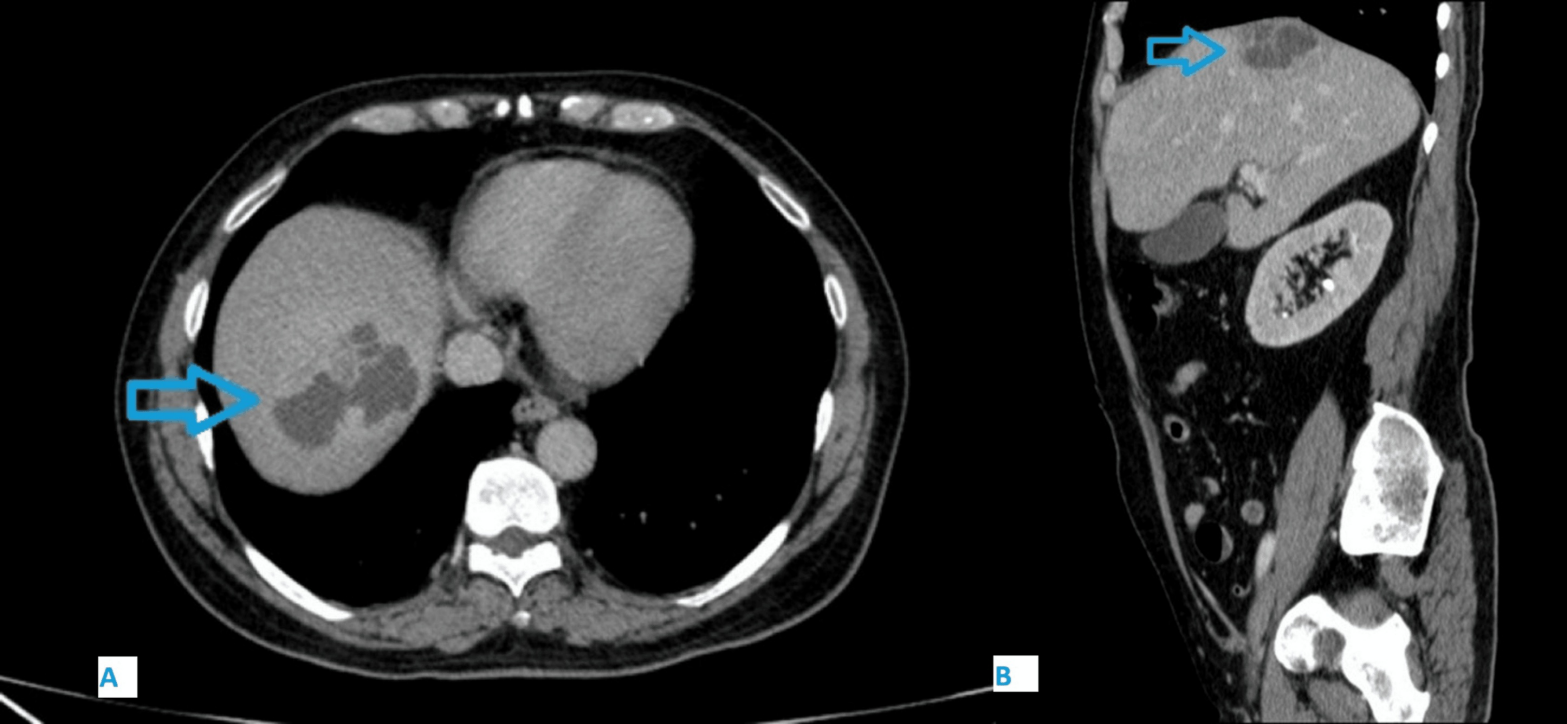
Abdominal CT images showing a liver lesion. (A) Axial view and (B) sagittal view CT of the abdomen reveals an irregular fluid-density lesion within the liver, demonstrating rim enhancement and a thin outer ring of hypoattenuation, suggestive of a liver abscess (indicated by arrows).
